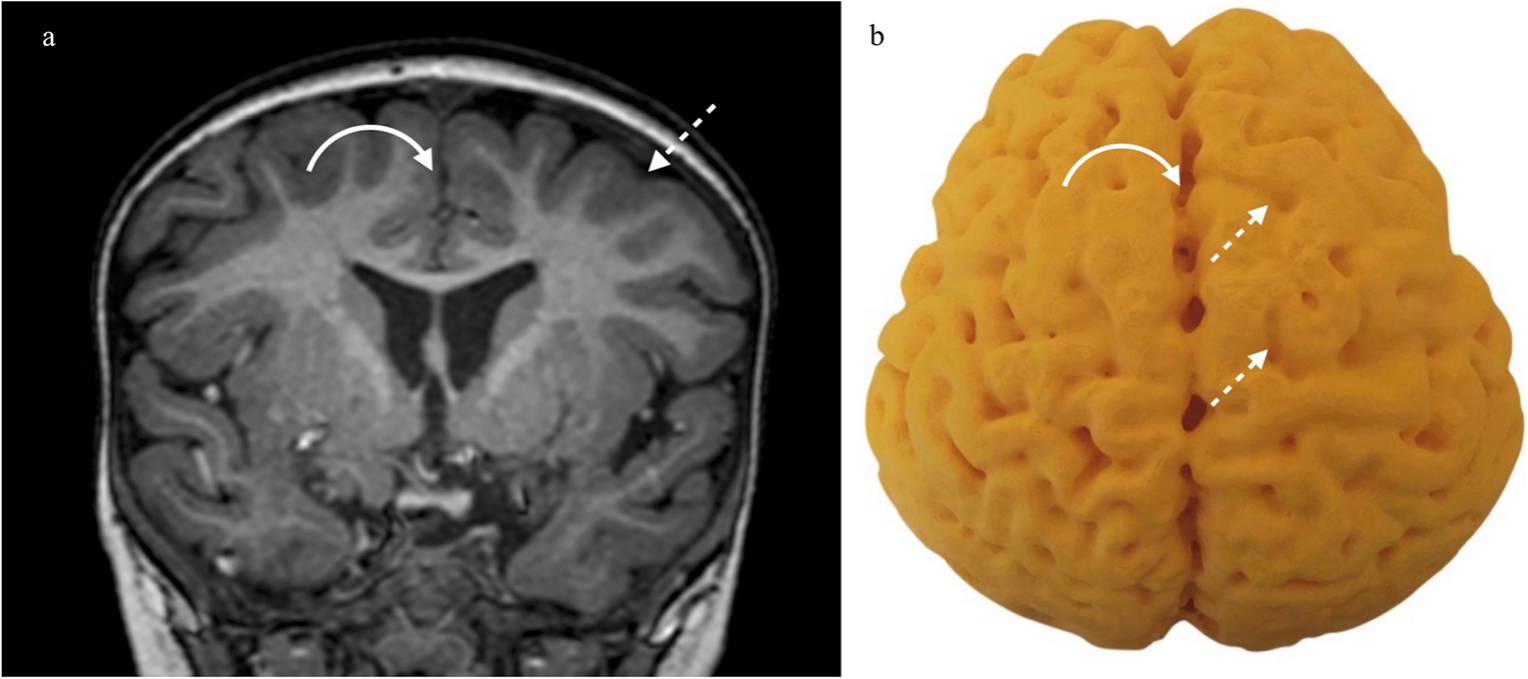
a, b Control patient with ‘normal’ MR imaging at the age of 4 years and accompanying 3D print. a Coronal T1 weighted MRI at the level of the 3rd ventricle in a healthy child age-matched with those in Figs. 3 and 4. The interhemispheric fissure is narrow (curved arrow) and the hemispheres and gyri are closely apposed (dashed arrow). b 3D printed model of the brain. The interhemispheric fissure is narrow (curved arrow) and the hemispheres and gyri are closely apposed (dashed arrows)
